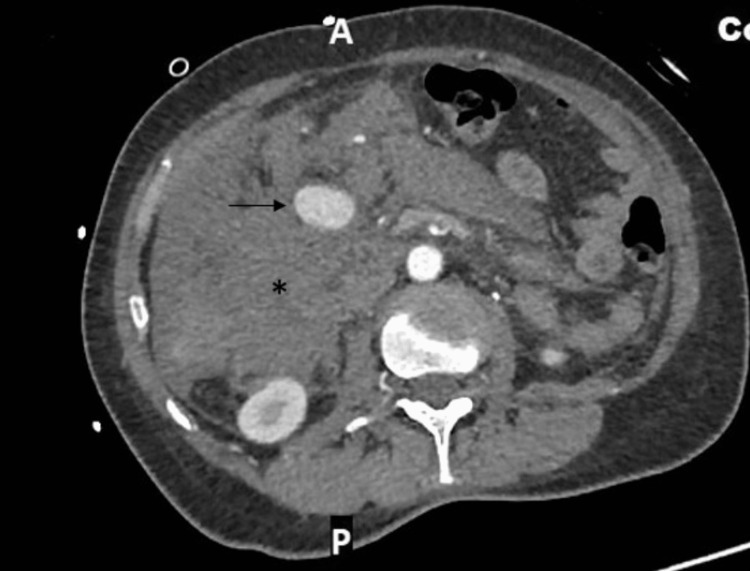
Arterial phase CT scan. Axial image demonstrating haemoperitoneum (*) and large pseudoaneurysm (thin arrow)
